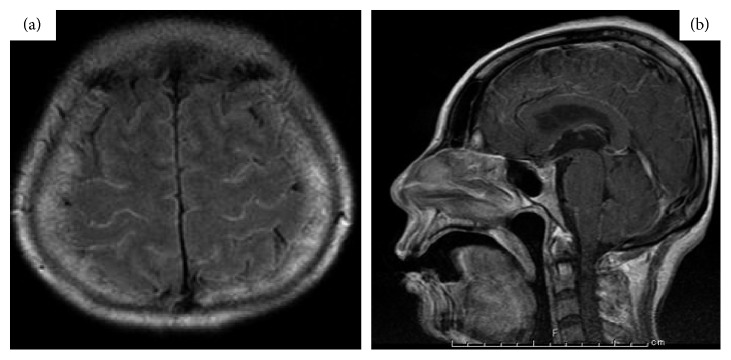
(a, b) Fluid-attenuated inversion recovery (FLAIR) magnetic resonance imaging of the brain revealed pia-subarachnoid enhancement (case 3).
